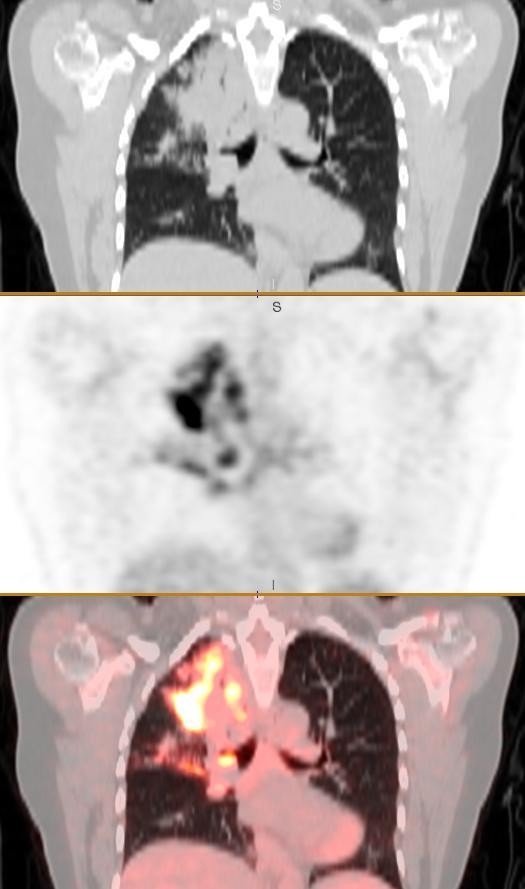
Coronal series showing dense consolidation with areas of increased uptake in the right upper lobe: CT, positron emission tomography and fused PET/CT images.
